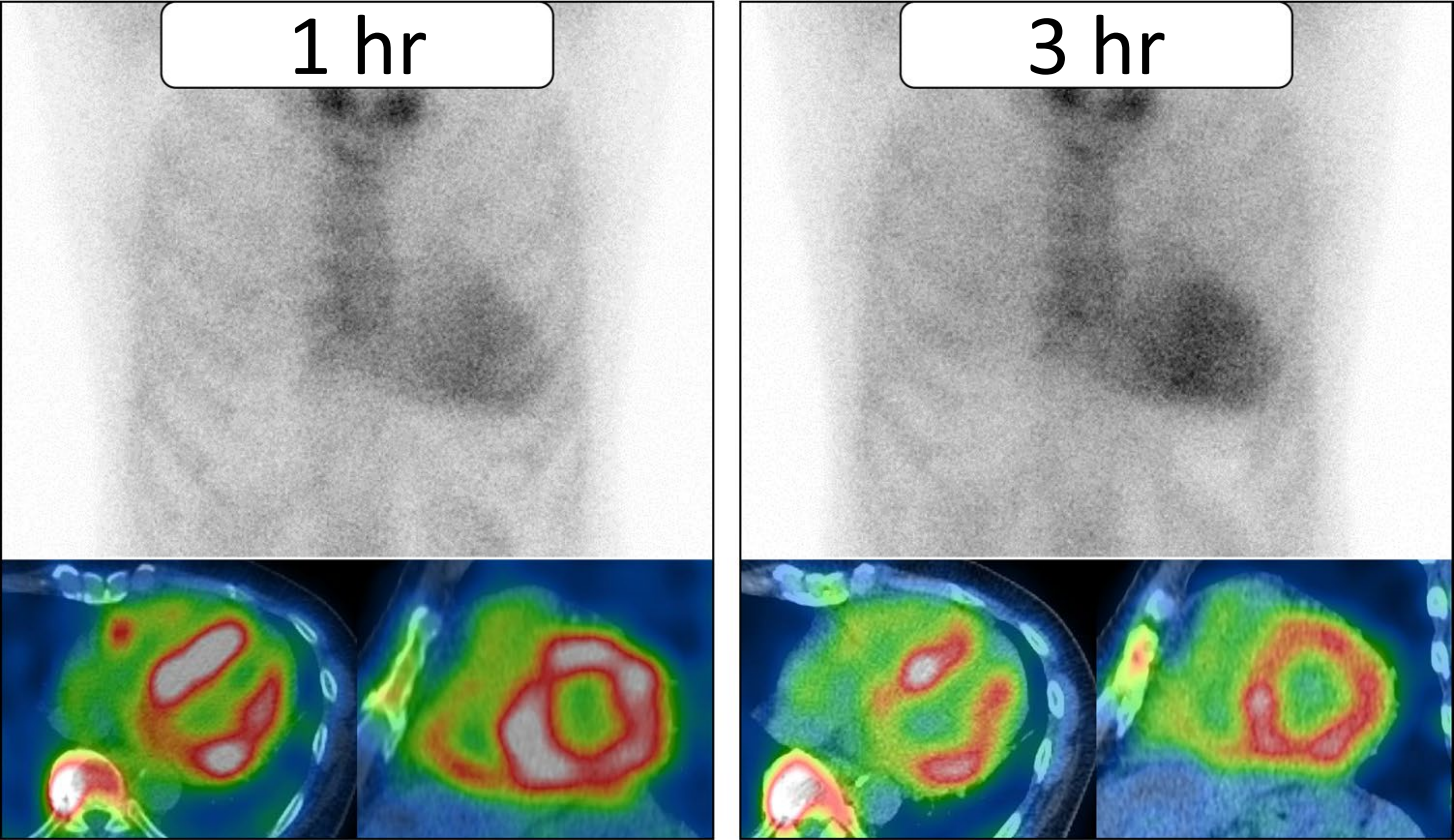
Representative case of transthyretin cardiac amyloidosis in a man in his 70 s. Planar images acquired at 1 and 3 h post-injection of ^99m^Tc-pyrophosphate (PYP), along with corresponding axial and short-axis SPECT/CT images, are shown. There is intense radiotracer uptake in the left ventricular myocardium, with relatively reduced uptake in the apical region. Mild uptake is also observed in the right ventricle on SPECT images. Visual assessment corresponds to Grade 3 (uptake greater than rib uptake with mild rib uptake). Quantitative evaluation yielded a heart-to-contralateral lung ratio of 1.88 at 1 h and 1.78 at 3 h
